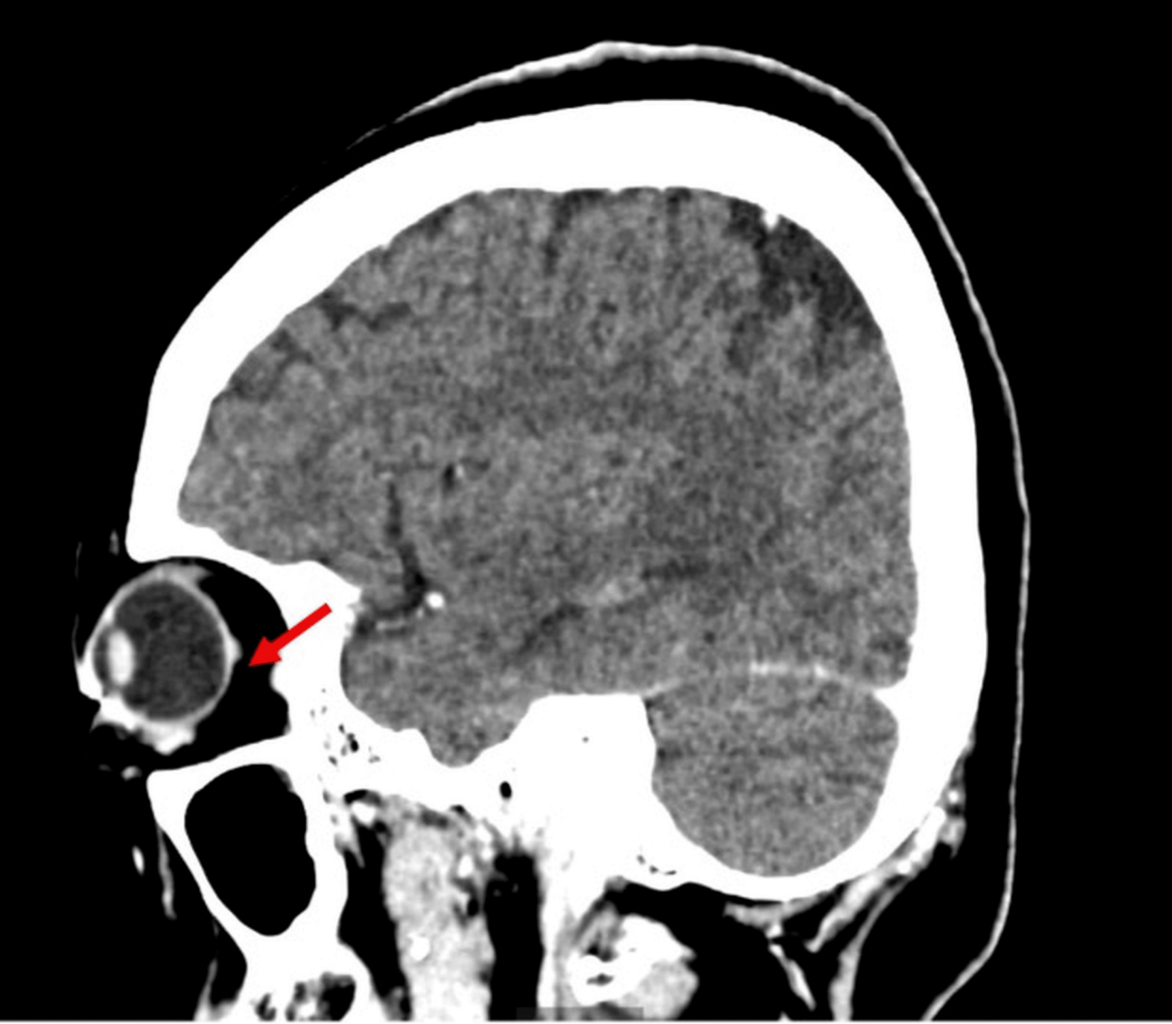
Follow-up CT showing regression of the left choroidal lesion (red arrow indicates the same area as in Figure 2).
